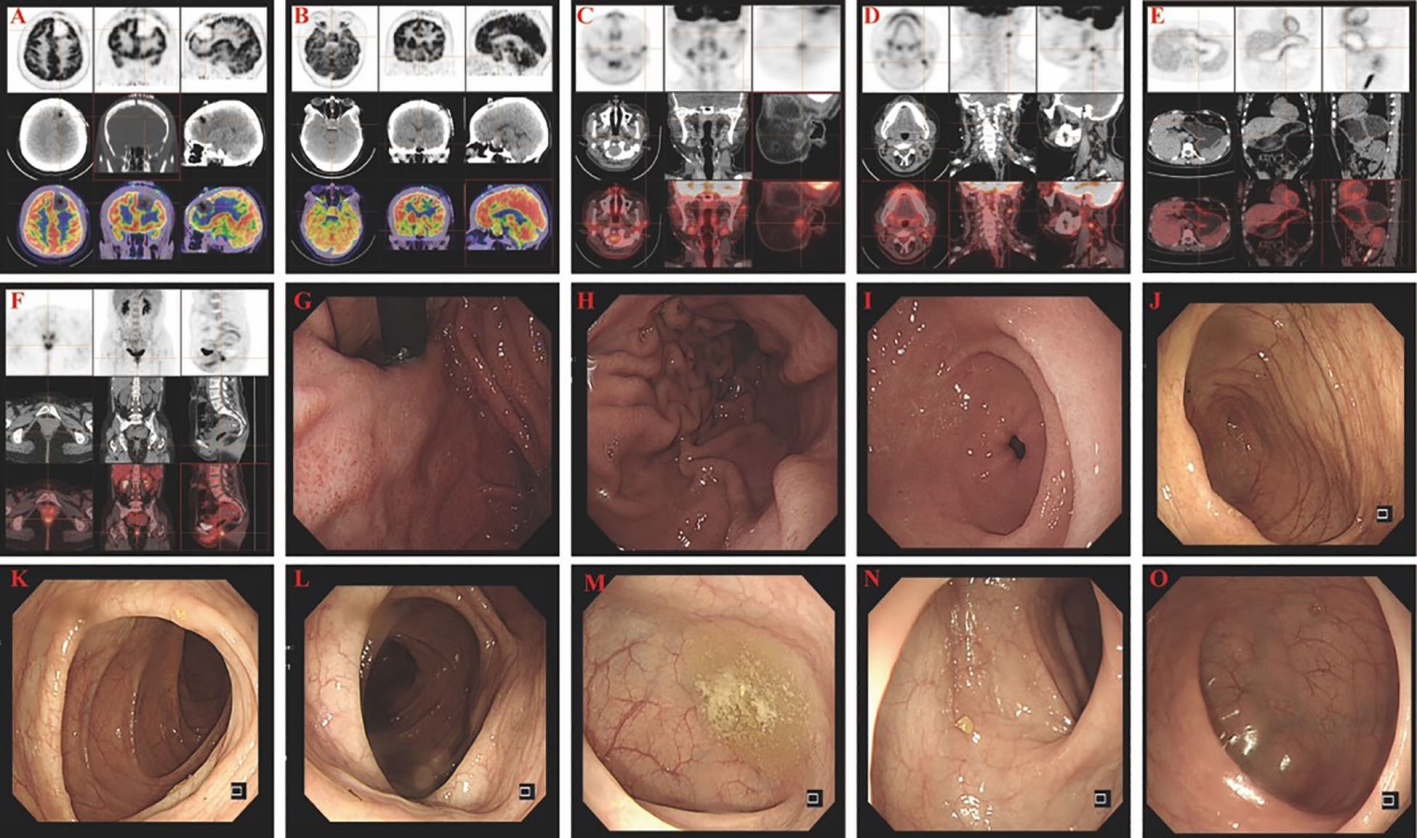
PET-CT, upper gastrointestinal endoscopy, and colonoscopy findings. (A) PET-CT image showing increased FDG uptake at the left frontal scalp surgical site (SUVmax: 12.6), consistent with postoperative inflammation. The left frontal lobe resection cavity demonstrates postoperative changes with hypometabolic areas suggestive of hemorrhage, fluid collection, and perifocal edema, resulting in compression of the left frontal horn and adjacent sulcal effacement. (B) A hypermetabolic lesion in the sellar region (27 × 19 × 25 mm) protruding into the suprasellar cistern and third ventricle, with increased FDG uptake (SUVmax: 12.4), causing mild third ventricular compression. (C) A left parotid nodule (short axis ~8 mm) with mildly increased FDG uptake (SUVmax: 3.3). (D) Multiple enlarged cervical lymph nodes are seen bilaterally in level Ib to III, with some showing increased FDG activity (SUVmax: 4.6); the largest is located in the left level II (short axis ~10 mm). (E) PET-CT image of the stomach shows uneven, diffuse FDG uptake (SUVmax: 3.9) without obvious wall thickening, consistent with chronic gastritis. (F) Increased FDG uptake is observed in the anorectal region and sigmoid colon (SUVmax: 3.4–5.3), although the perirectal fat planes remain preserved. (G–I) Upper gastrointestinal endoscopy reveals chronic atrophic gastritis involving the gastric fundus (G), body (H), and antrum (I), with no evidence of ulceration or malignancy. (J–O) Colonoscopic examination of the ileocecal region (J), ascending colon (K), transverse colon (L), descending colon (M), sigmoid colon (N), and rectum (O) shows no mucosal lesions or neoplastic abnormalities.
